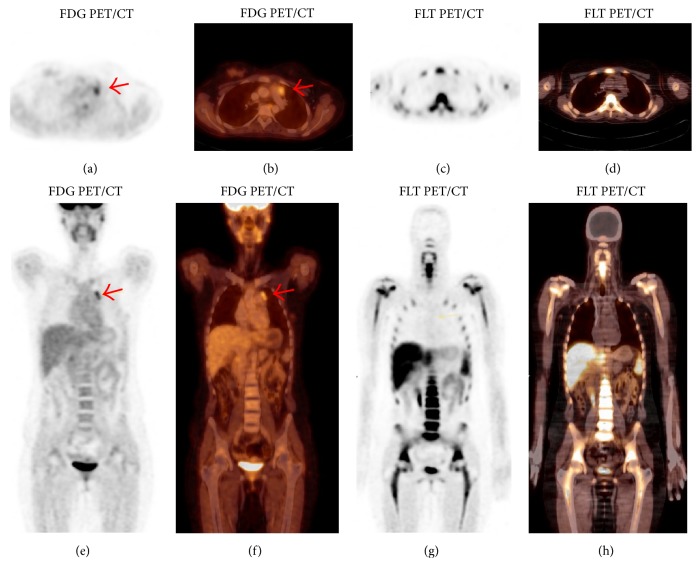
18-year-old female with stage II Hodgkin lymphoma (patient 4) demonstrating increased uptake in a left prevascular lymph node on FDG PET/CT (a, b and e, f) with 18F-FDG SUV 3.0 and no uptake on 18F-FLT/CT (c, d and g, h). Follow-up 18F-FDG PET/CT performed three (3) months later (not shown) again showed evidence of increased metabolic activity in the prevascular region in the upper mediastinum with SUV of 3.1 and not significantly changed compared to the previous study, and no other focus of increased 18F-FDG uptake to suggest disease progression.
